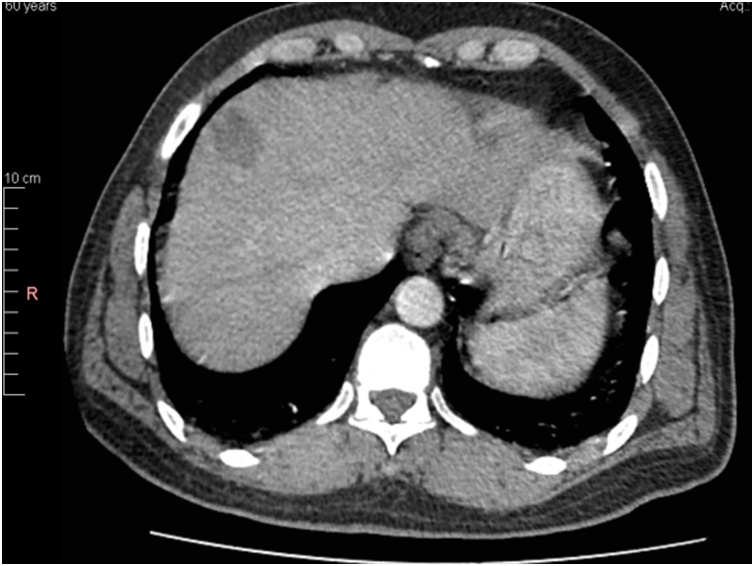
CT scan showing single liver lesion.
